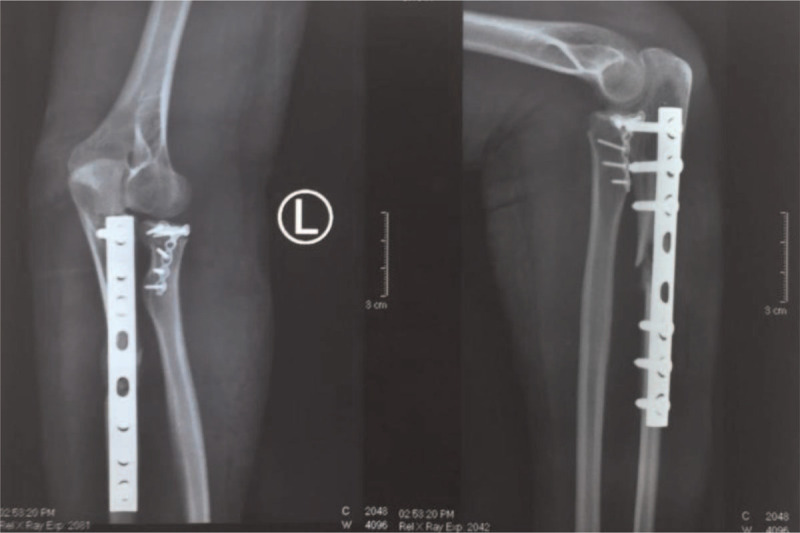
The plain radiograph of the patient's left forearm after open reduction and internal fixation, anteroposterior (AP) and lateral (L) view.
